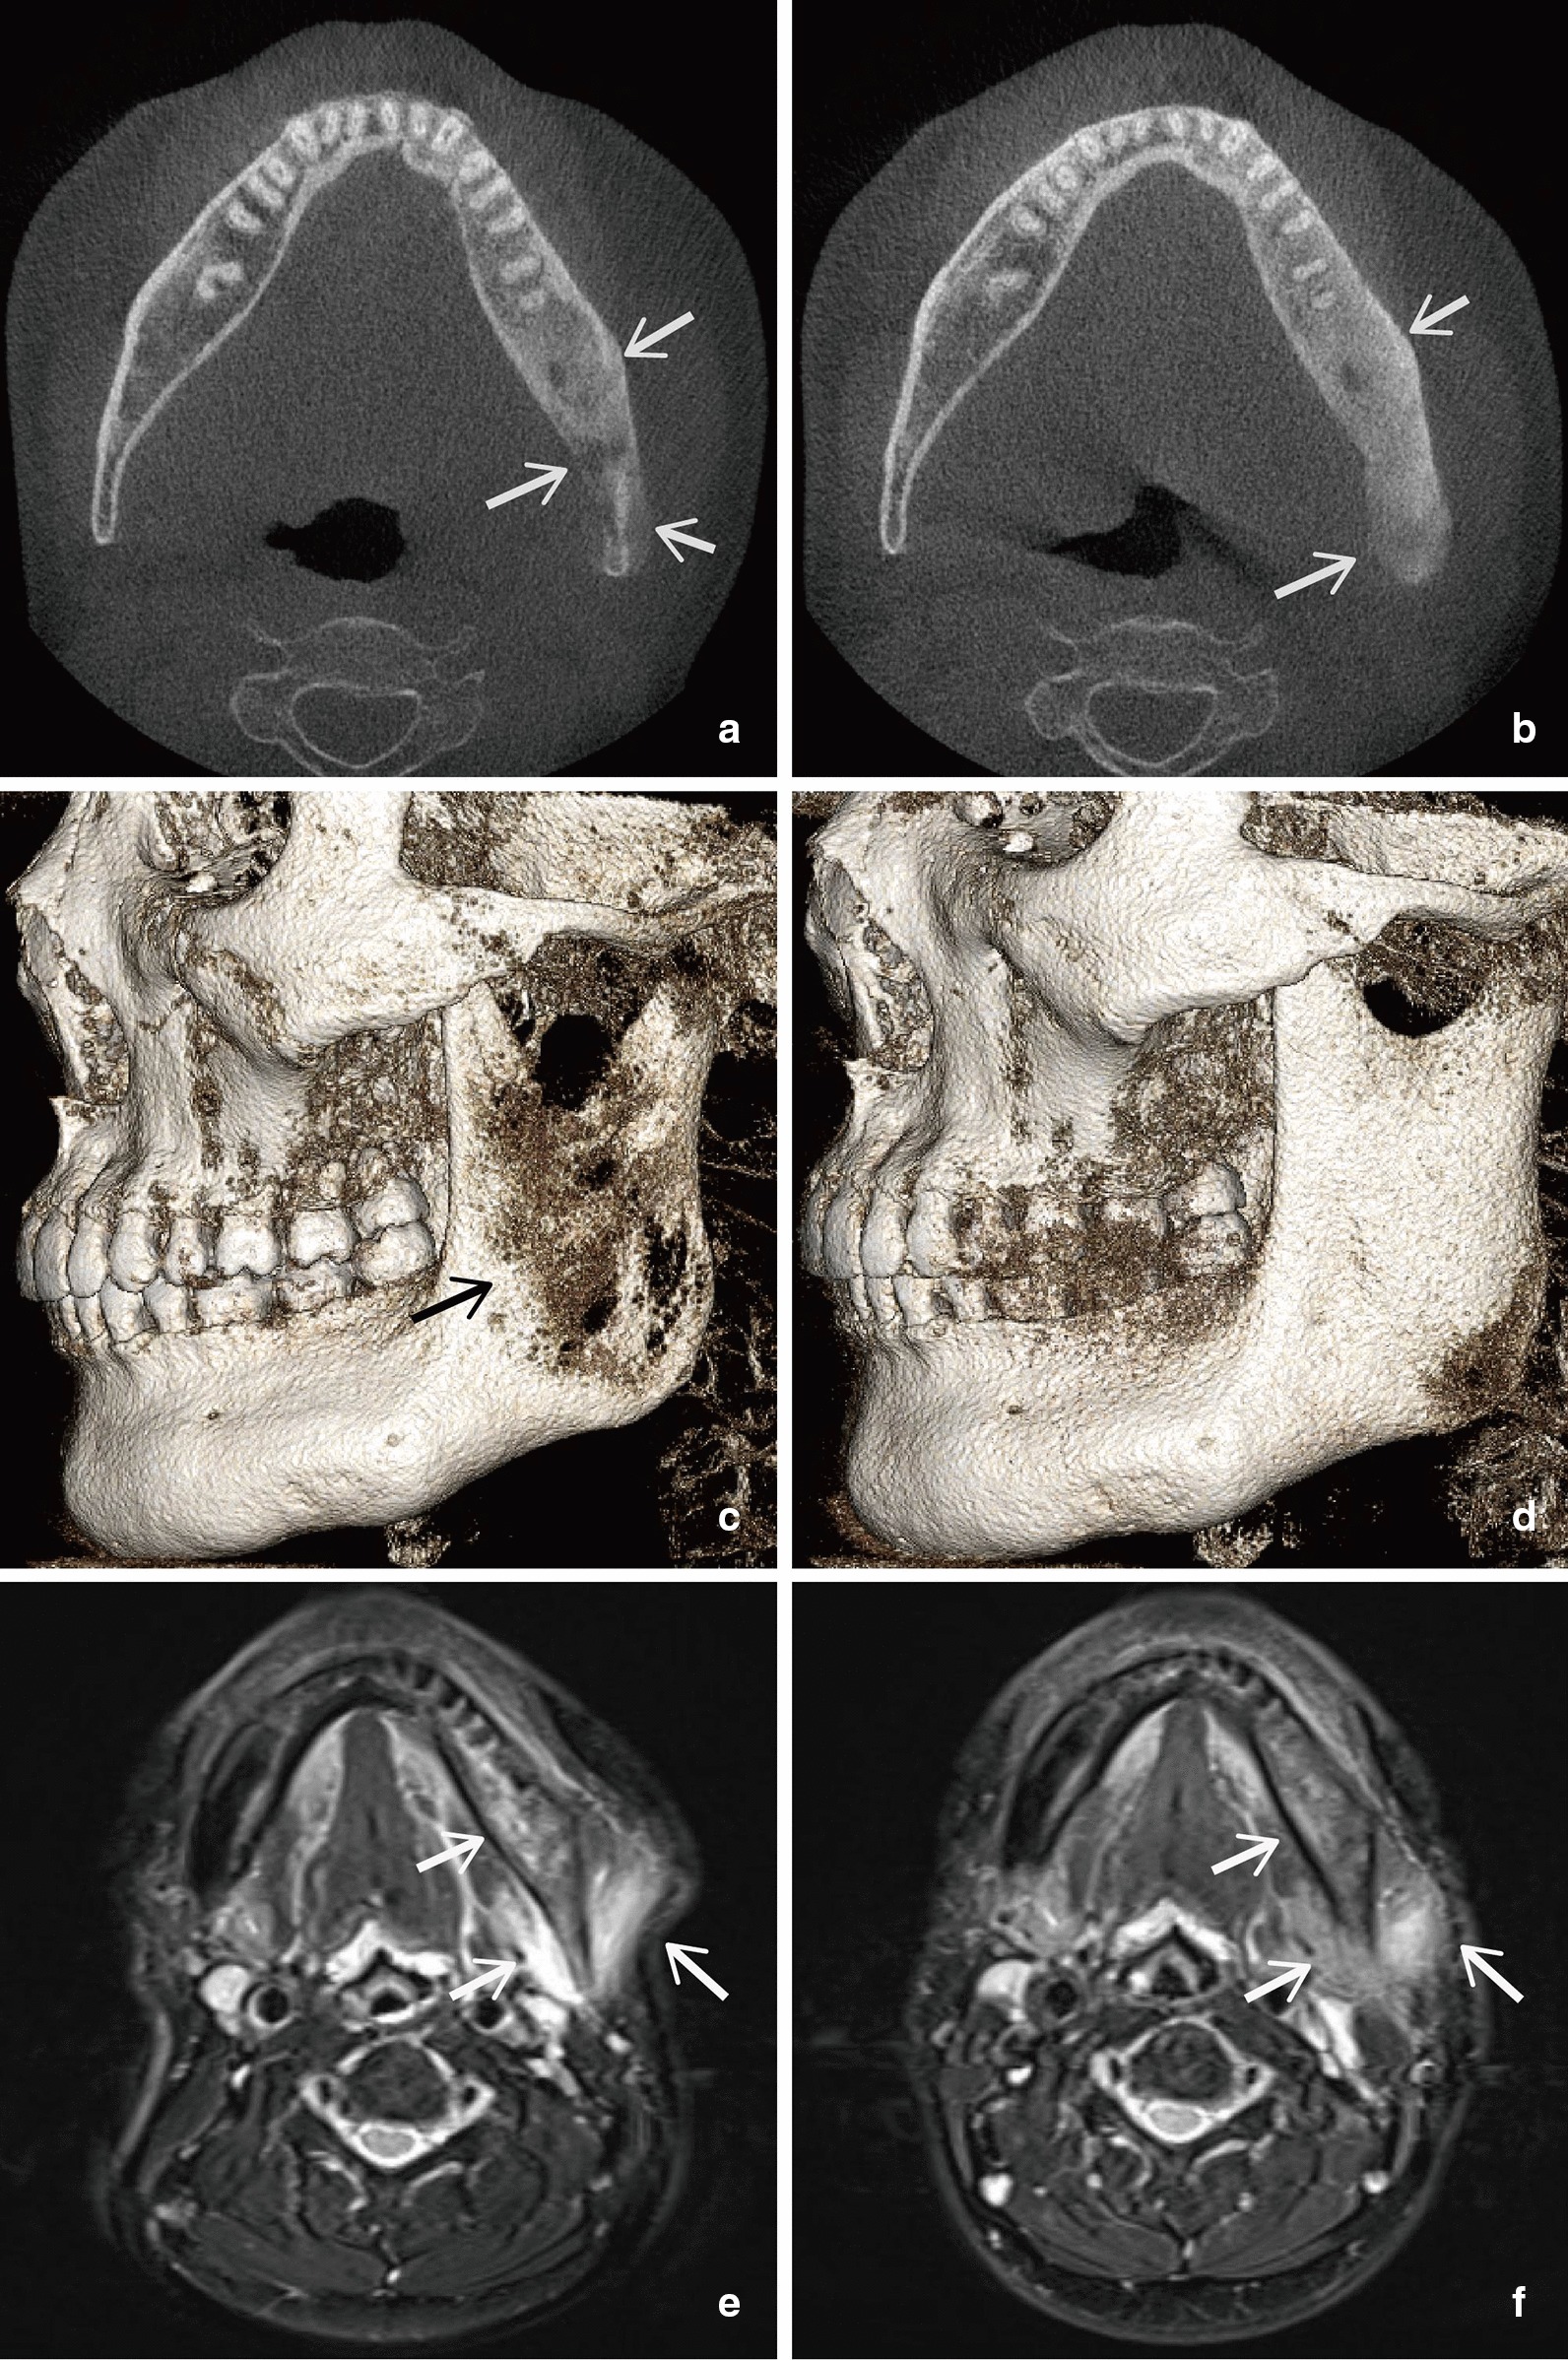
Mandible CBCT axial view and 3D reconstruction of a TNF-α inhibitor resistant patient (a, c) at baseline, showing diffuse lysis, sclerosis, and subperiosteal bone formation (b, d), and 1 year after bisphosphonate treatment, showing mainly sclerosis and hyperostosis. Fat Suppression T2-Weighted MR imaging (e) at baseline, showing oedema of bone marrow and masticatory muscles, and f 1 year after bisphosphonate treatment, showing alleviation of oedema
